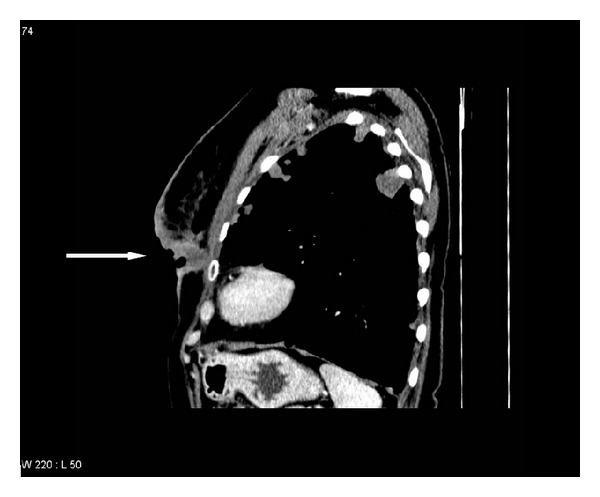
Sagittal computed tomography scan of the chest showing local relapse in the lower inner quadrant of the breast after breast conserving surgery and adjuvant treatment.
